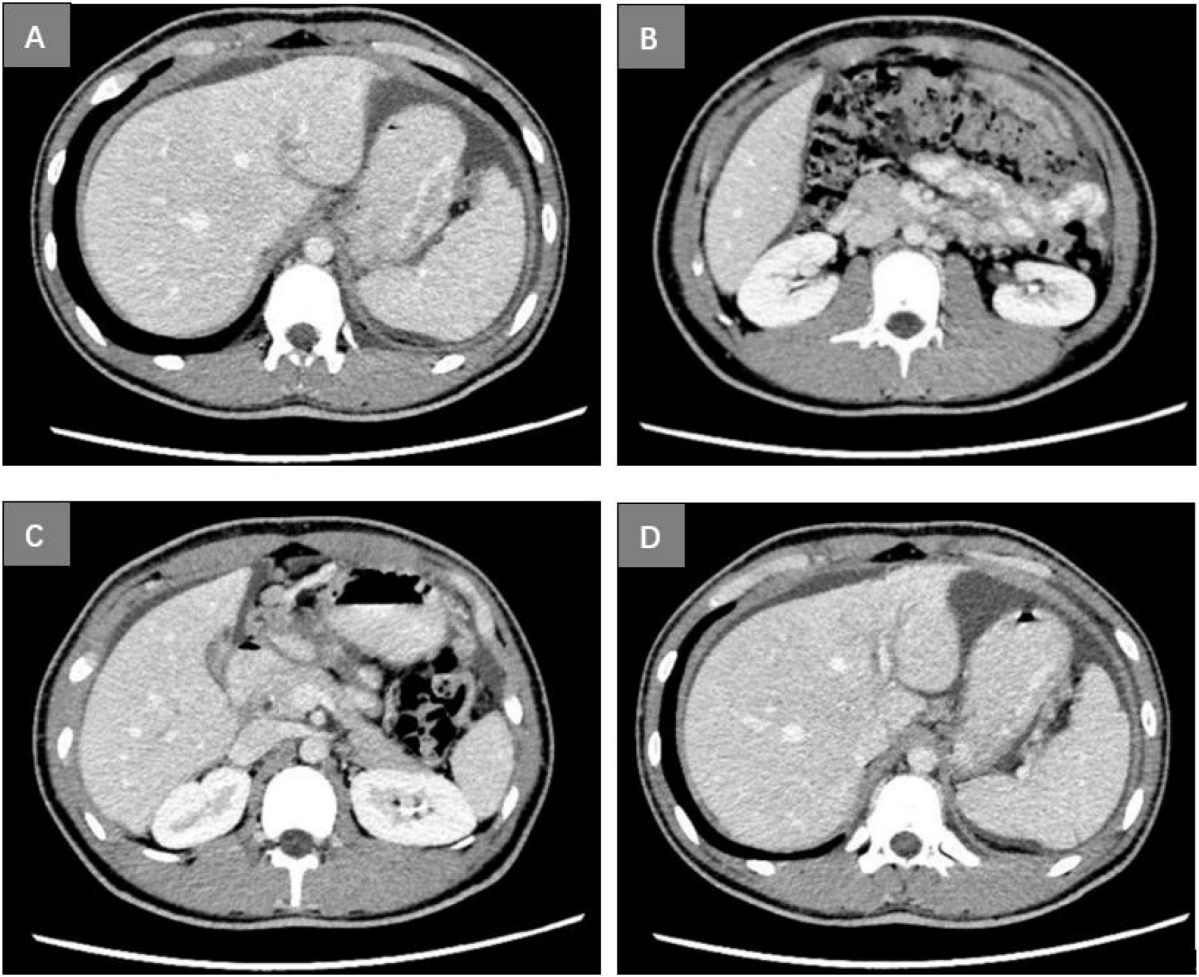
The CT image of the proband patient: the thickening of the gastric wall on the greater curvature side, with a thicker area measuring approximately 2.1 cm, and uneven enhancement on the contrast-enhanced scan (A) nodular or pancake-like thickening and enhancement of the peritoneum (B) one enlarged lymph node can be noticed around the stomach, with enhancement visible on the contrast-enhanced scan, measuring approximately 1.0 cm in diameter (C) free fluid-like density shadow was observed in the abdominal cavity (D).
